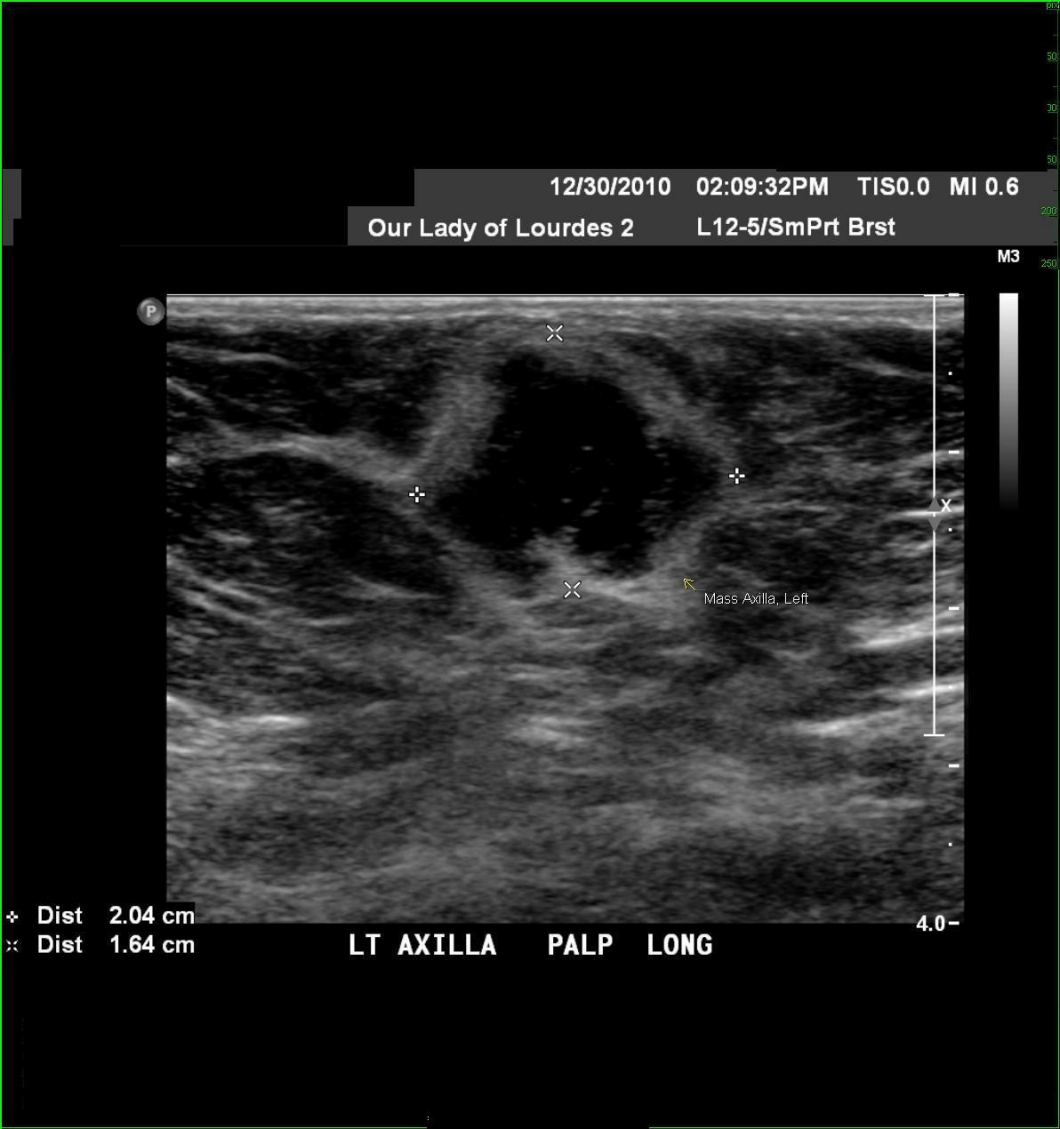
Ultrasound image of the irregular mass in the left axilla.
